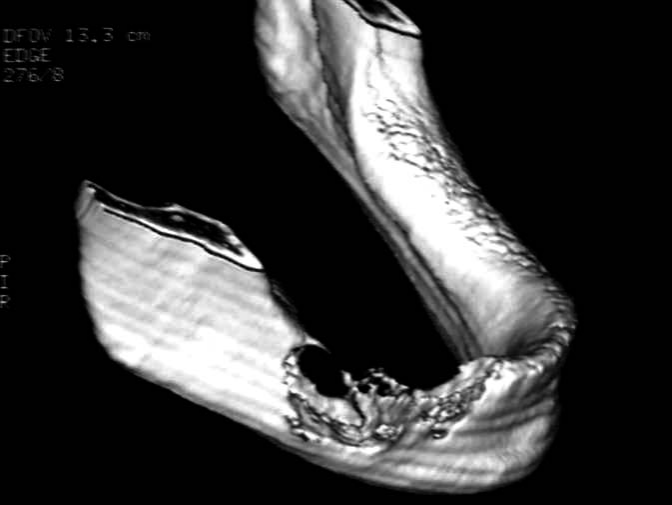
3D CT of the external aspect of the jaw shows an apparently infiltrative nature of the lesion with invasive margins involving contiguous healthy bone.
